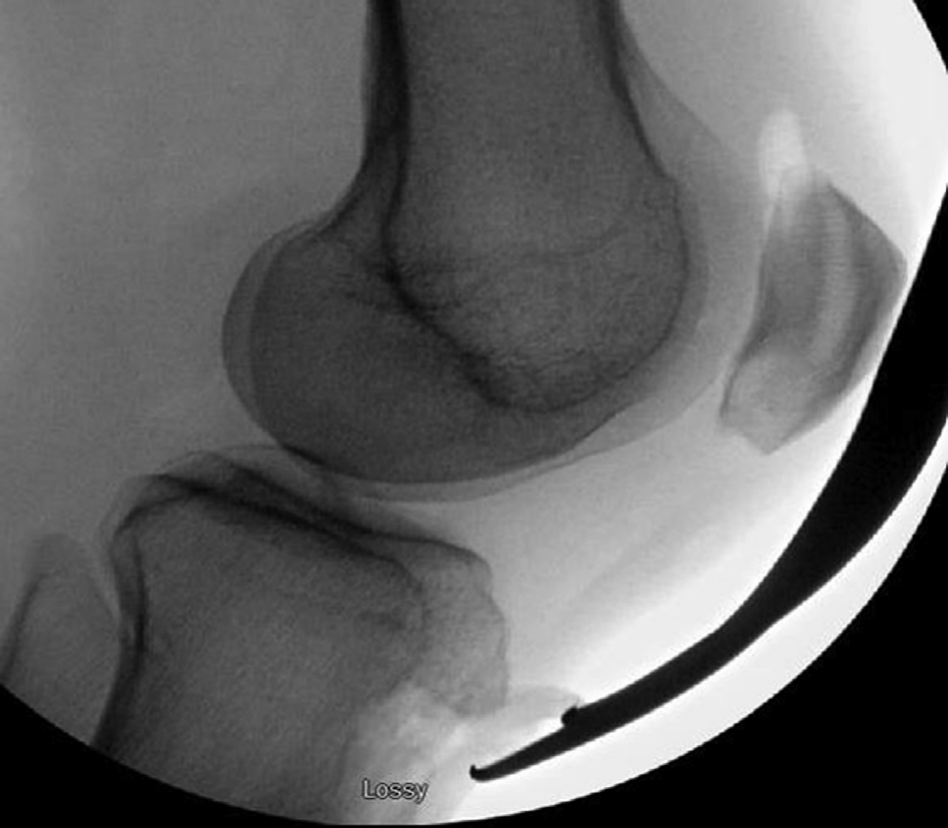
Intraoperative lateral X-ray fluoroscopic image of a left knee after tibial tubercle distalization osteotomy to confirm that the tubercle is appropriately positioned and patellar height is appropriate.
